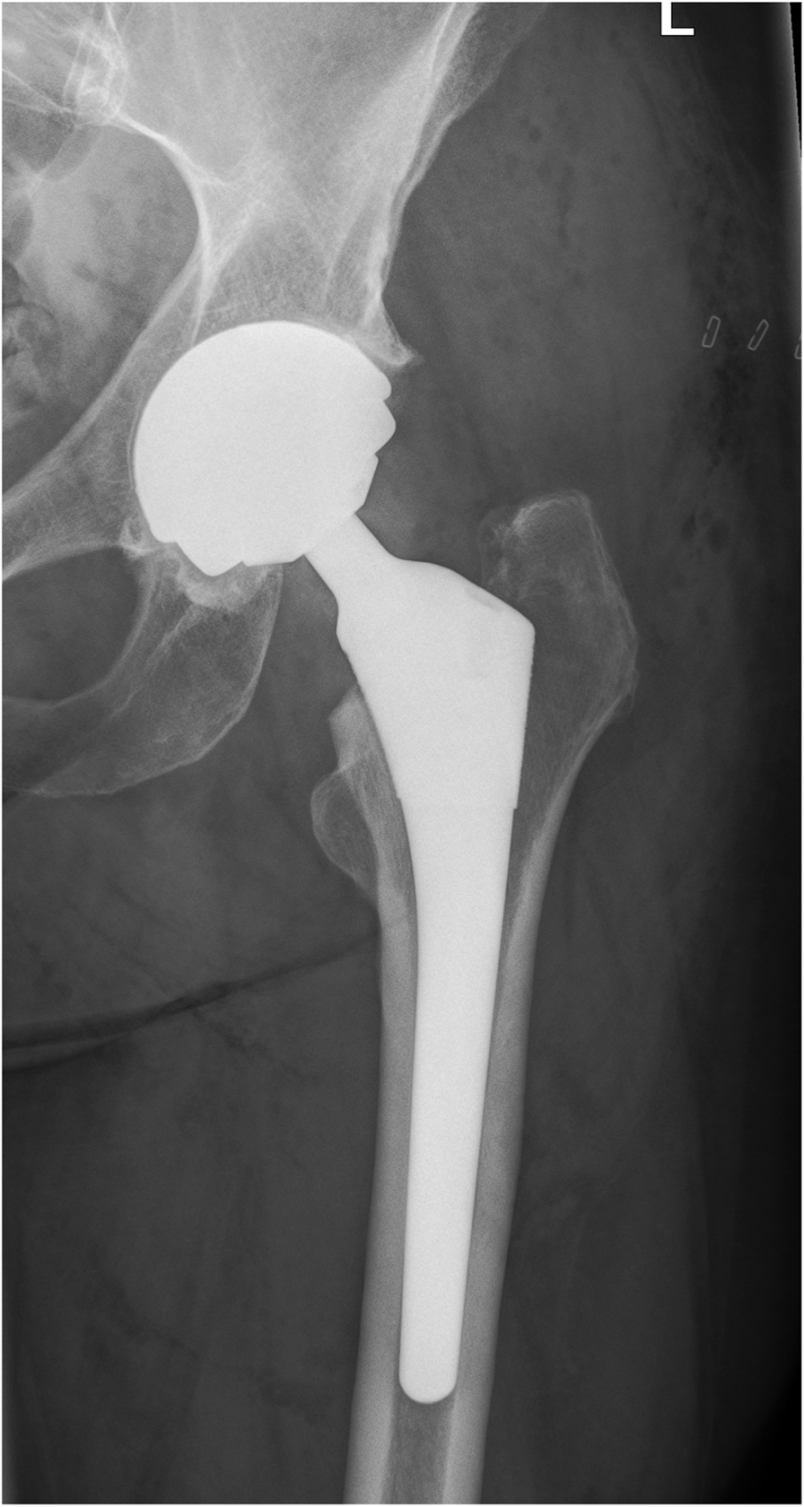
Postoperative x-ray (MoM-LDH-THA)
